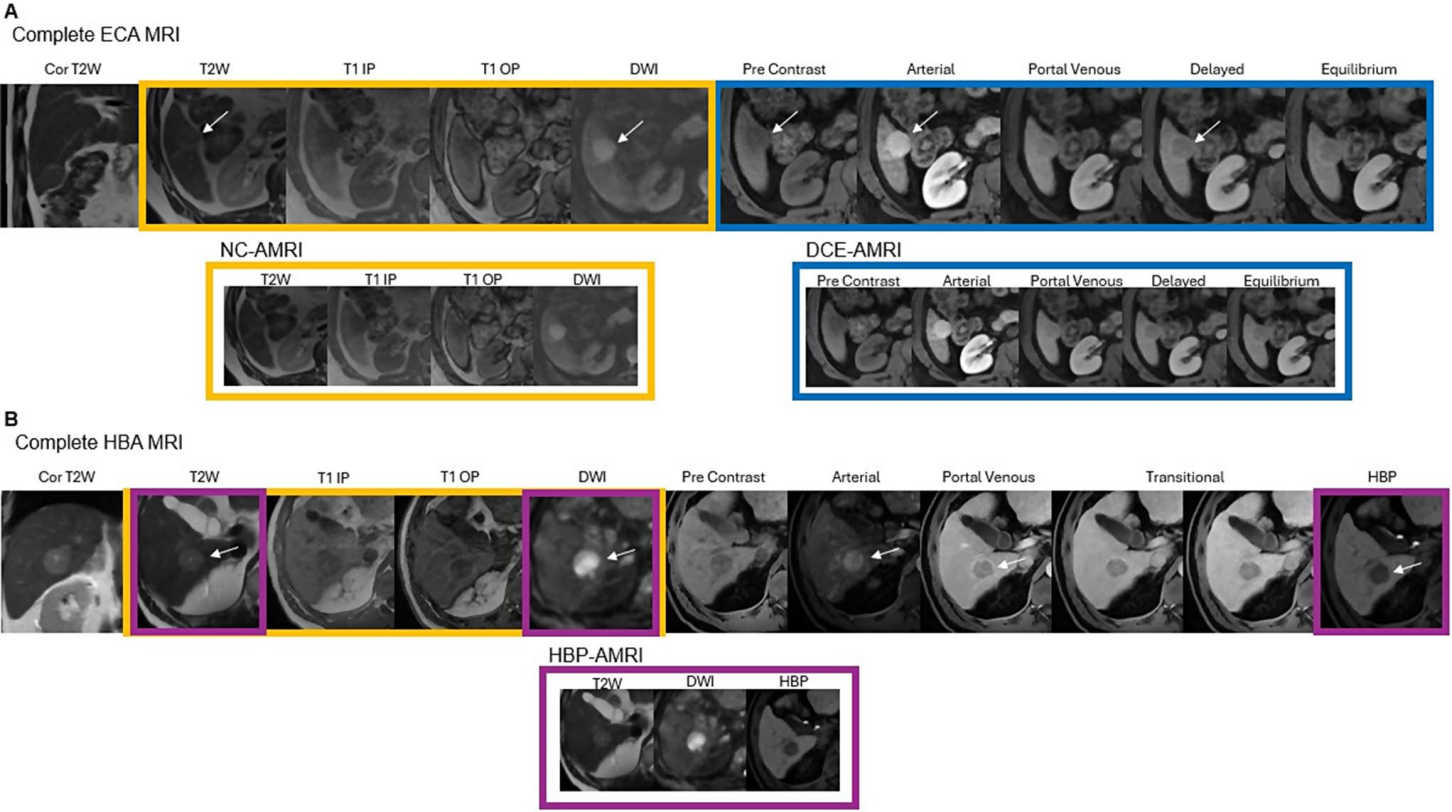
Sample multiphasic MRI and AMRI protocols in two patients with HCC. (A) Complete multiphasic MRI protocol utilizing an extracellular contrast agent and the select sequences utilized in the NC-AMRI and DCE-AMRI protocols. Images show a well circumscribed, partially exophytic mass (arrows) in hepatic segment 5 which is mildly T2 hyperintense, demonstrates restricted diffusion, and has arterial phase hyperenhancement, washout, and an enhancing capsule, diagnostic of HCC. (B) Complete multiphasic hepatobiliary agent (gadoxetic acid) enhanced MRI protocol and select sequences utilized in the HBP-AMRI protocol. Images show a well-circumscribed mass (arrows) in hepatic segment 6 which is mildly T2 hyperintense and demonstrates mild signal drop on the out-of-phase sequence as well as restricted diffusion. The mass also shows arterial phase hyperenhancement, washout, and corresponds to a hepatobiliary phase defect
